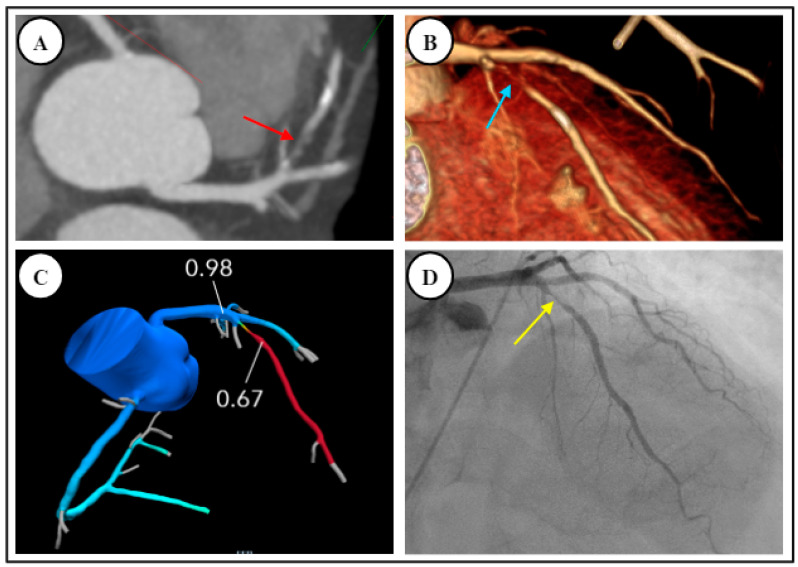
Obstructive coronary artery disease. A 79-year-old male presented to Emergency Room with two-week history of chest pain. (A,B) CCTA demonstrated severe stenosis with mixed plaque in left anterior descending artery (CAD-RADS = 4) on axial imaging, red arrow, and 3D reconstruction, light blue arrow, respectively. (C) FFR-CT positive for ischemia with a value of 0.67. (D) Invasive coronary angiography confirmed severe obstructive disease, yellow arrow; patient proceeded to percutaneous coronary intervention.
